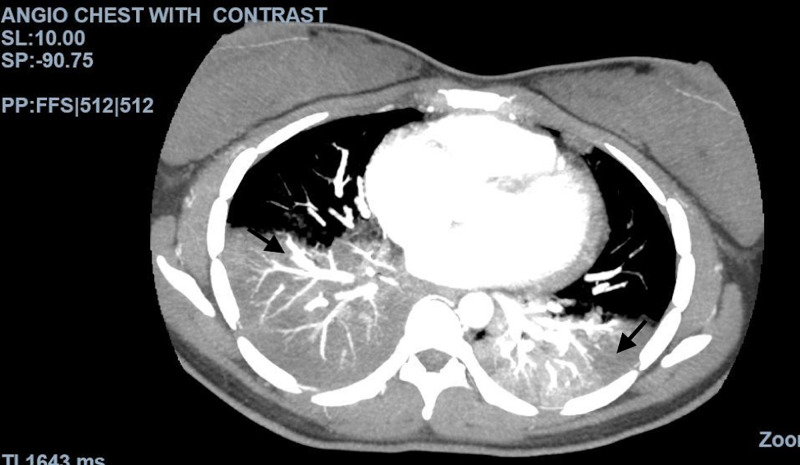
CT angiogram of the chest with contrast The image shows multifocal consolidation predominately involving the lower lobes, characteristic of pneumonia seen in COVID-19 CT: computed tomography; COVID-19: coronavirus disease 2019
